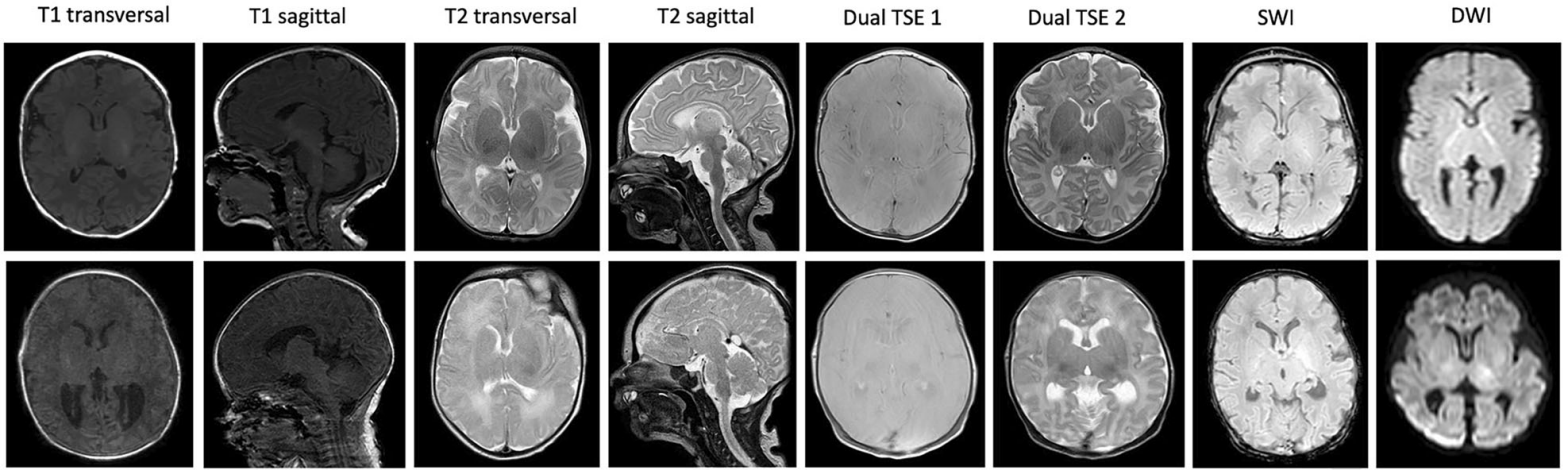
An overview of all applied sequences is presented in the upper row using the recently developed 16-channel radiofrequency receiving head coil MR-compatible incubator and in the lower row without it. From left to right, the sequences include T1 transversal, T1 sagittal, T2 transversal, T2 sagittal, Dual TSE 1 and 2, dual turbo spin echo in both T1 and T2 weighting, as well as the SWI sequence and the diffusion-weighted imaging (DWI) sequence; TSE, Turbo spin echo; SWI, susceptibility-weighted imaging
